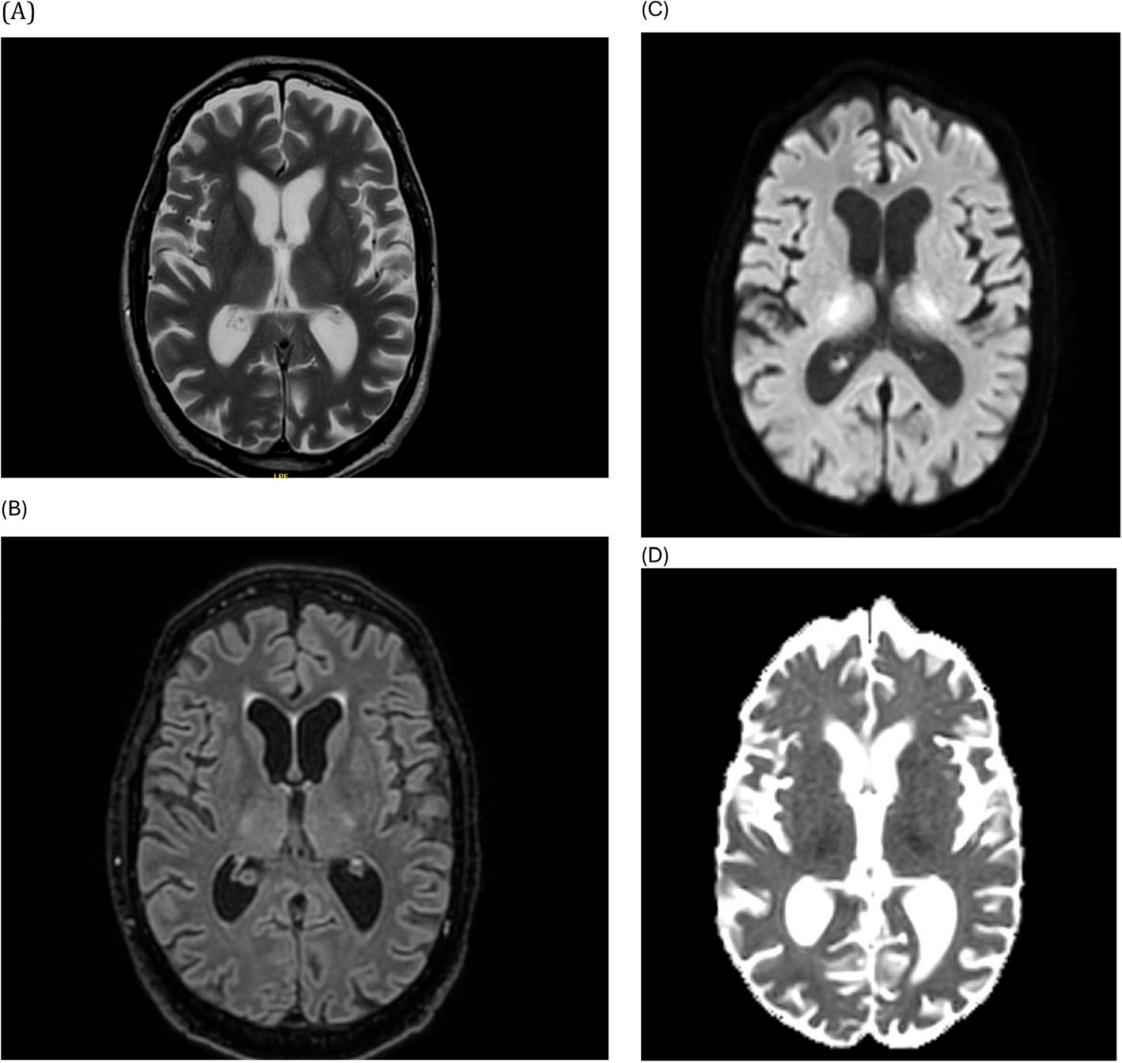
Brain MRI at presentation showing bilateral thalamic lesions: axial T2-weighted (A), FLAIR (B), DWI (C) and corresponding ADC map (D). Lesions demonstrate diffusion restriction with ADC hypointensity and no contrast enhancement
